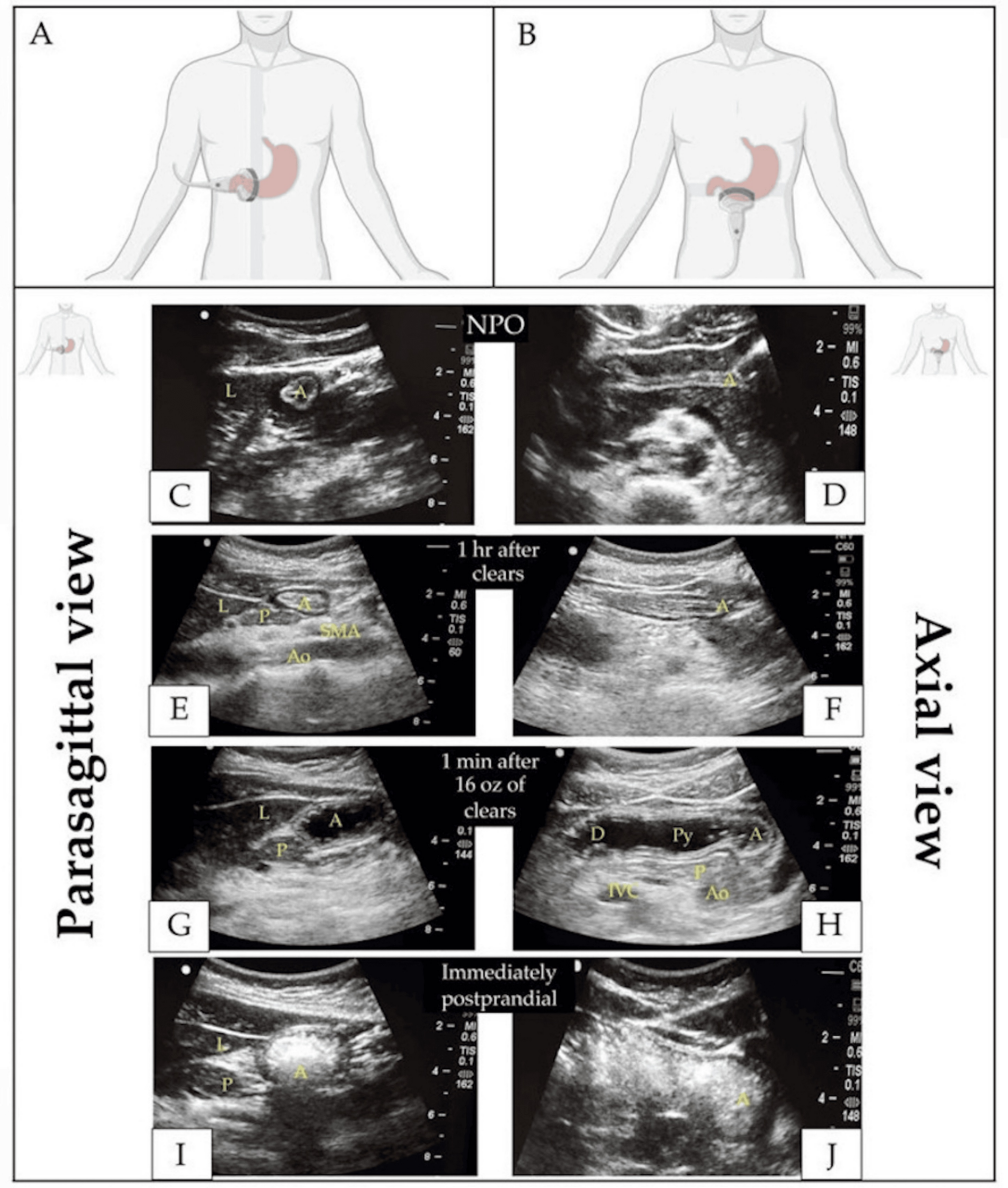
Gastric ultrasound for evaluation of gastric contents (A, B) Ultrasound probe location and orientation for parasagittal and axial views of the gastric antrum. Parasagittal (C, E, G, I) and axial (D, F, H, J) views of the gastric antrum: empty stomach (C, D); one hour after ingestion of clear liquids (E, F); one minute after drinking approximately 500 mL (16 oz) of clear liquids, showing an expanded lumen with hypoechoic contents (G, H); immediately after a meal with a characteristic “ground-glass” appearance (I, J). A: antrum; Ao: aorta; D: duodenum; IVC: inferior vena cava; L: liver; NPO: nil per os; P: pancreas; Py: pylorus; SMA: superior mesenteric artery Created with BioRender.com. Nguyen et al. (2023) [21]; Creative Commons Attribution (CC BY) license
